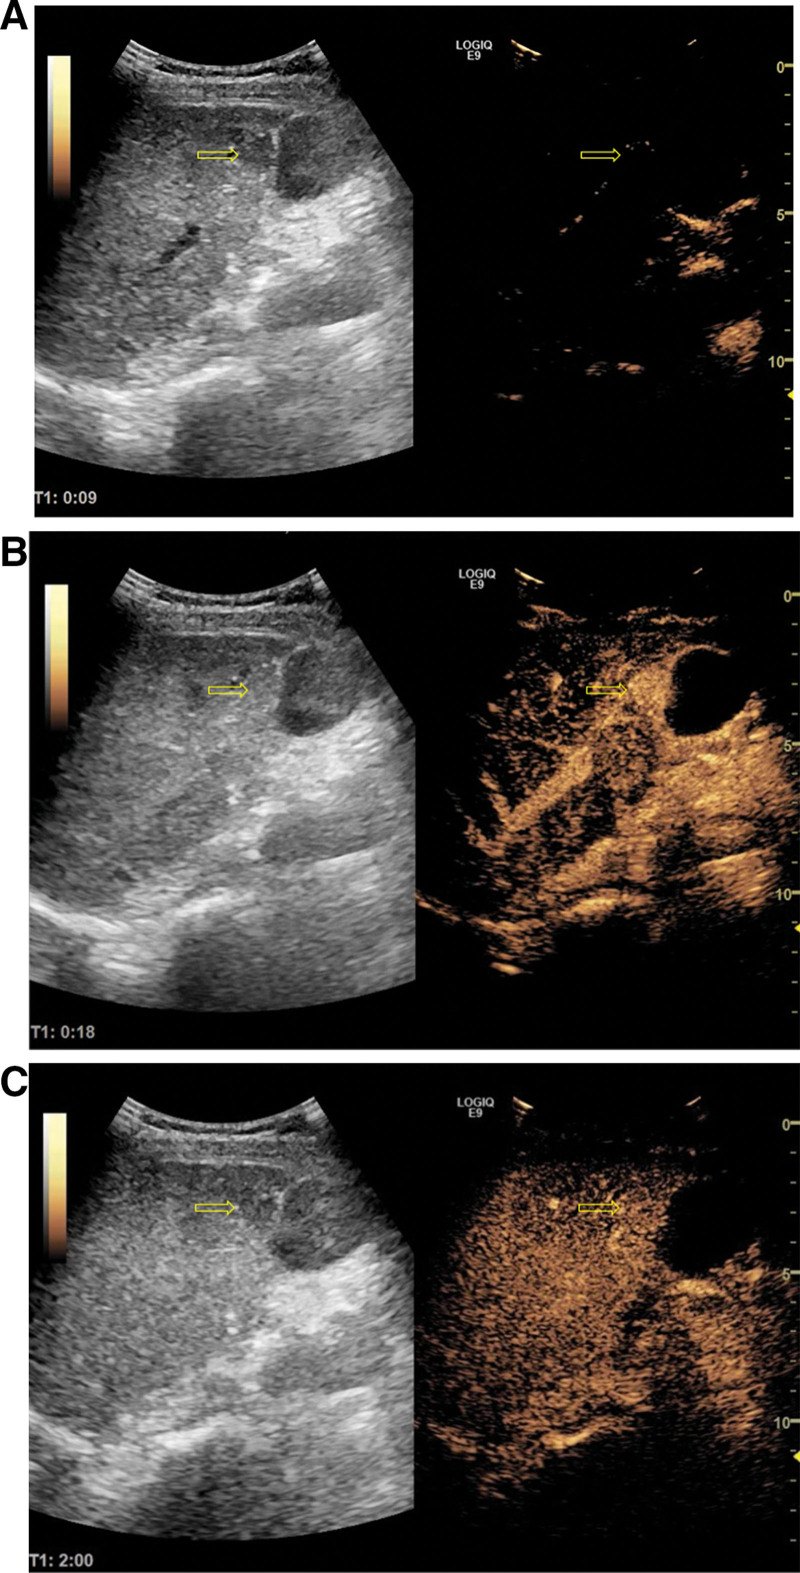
The results of CEUS in detection of hepatic nodules. (A) The results of arterial phase were examined by CEUS. (B) The arterial phase results of CEUS showed that the enhancement of hypoechoic nodules reached the peak at 17.2 seconds, which was much stronger than that of the hepatic parenchyma. (C) The results of CEUS showed that the hypoechoic nodules showed slightly higher enhancement in the delayed phase. CEUS = contrast-enhanced ultrasound.
